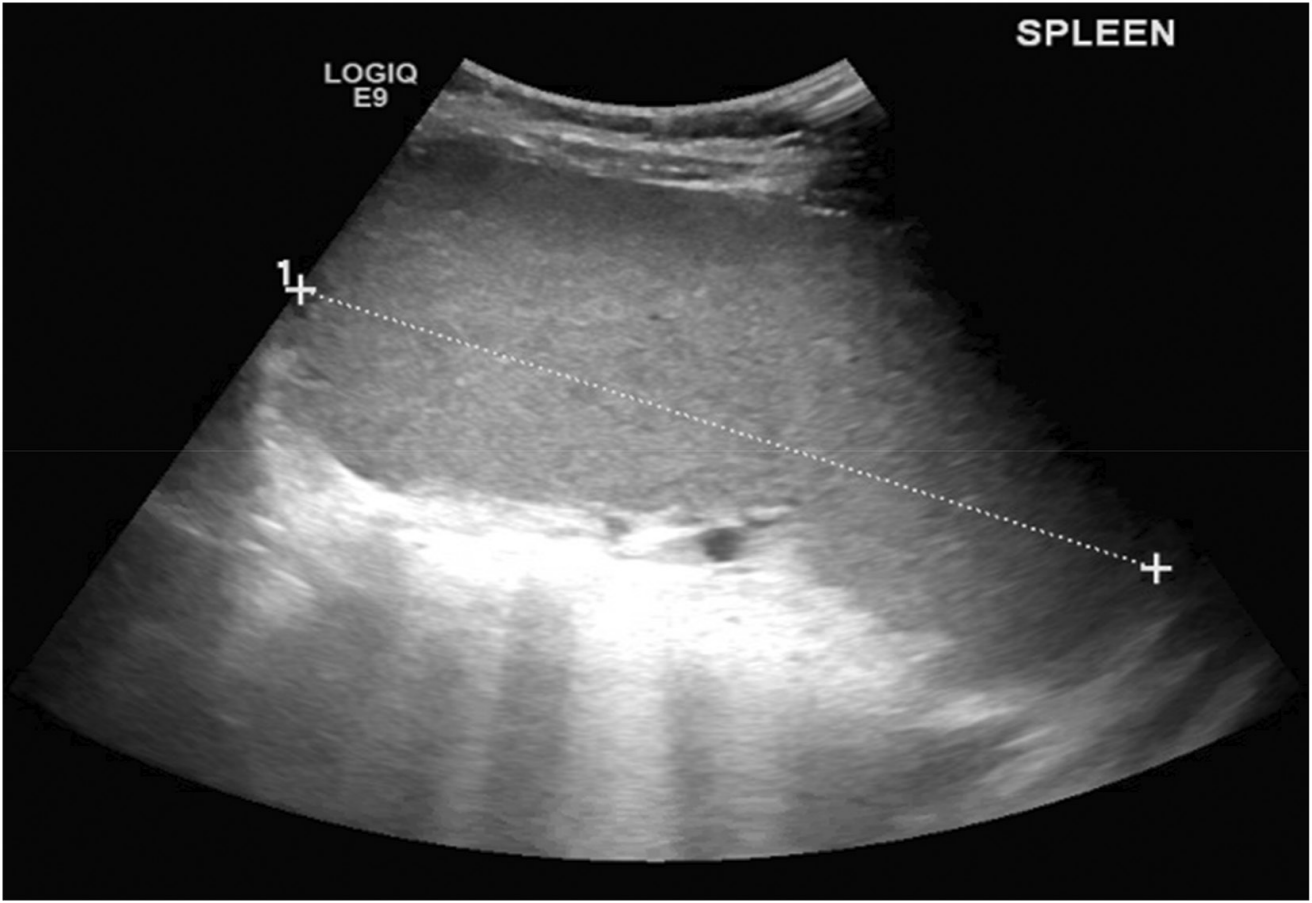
Image of a spleen on Ultrasound Abdomen. US showed spleen enlagrement with measured length of about 14.9 cm. (Refernce for Normal range for the patients age 6.5 ‐ 11 cm) and has a normal homogenous echotexture. No focal abnormality was displaed. No ascites seen.
